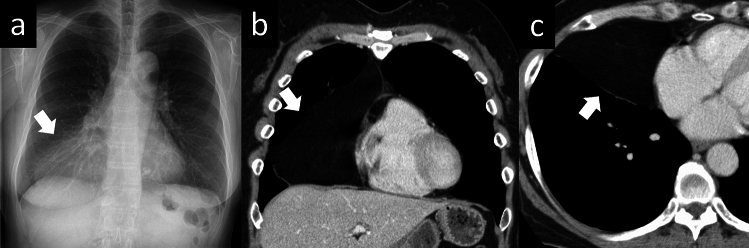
Thymolipoma. A 59-year-old male with thymolipoma. a Chest X-ray shows a unilateral ground-glass opacity in the right lower lung zone (arrow). b, c CT shows an adipose mass in the right hemithorax (arrow). CT, computed tomography
